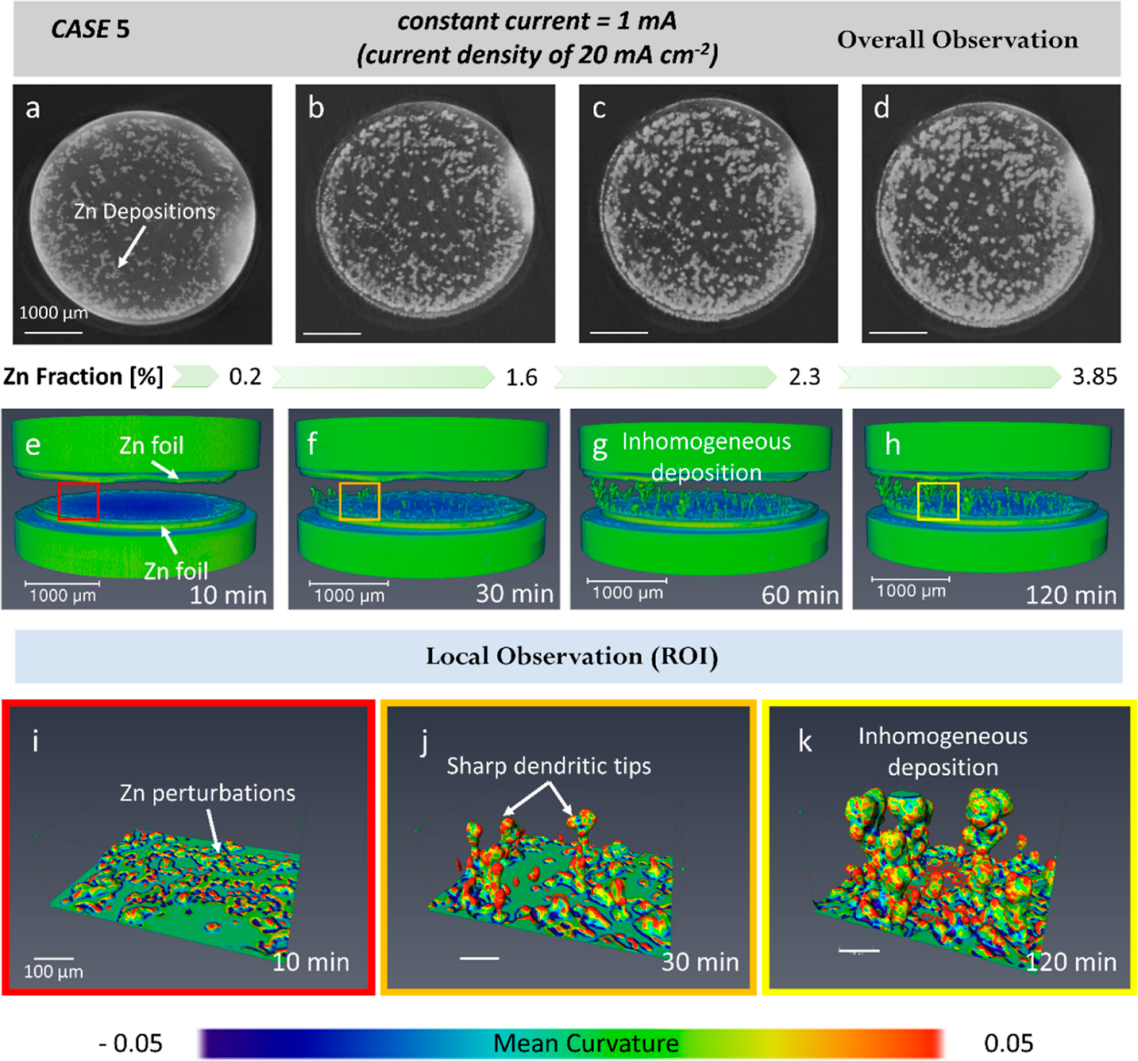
4D X-ray tomographic imaging of the fast zinc depositions
